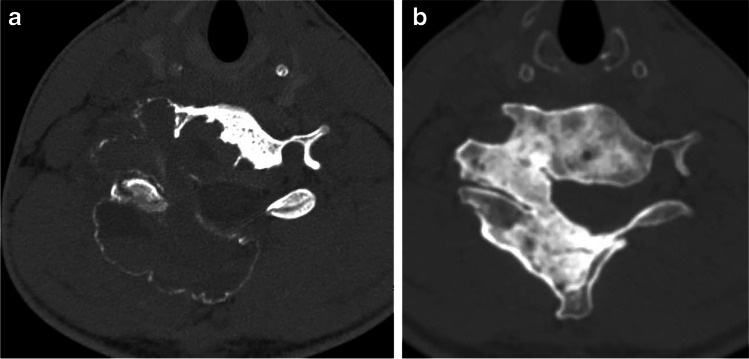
Computed tomography images in a 16-year-old boy (patient 5) with an aneurysmal bone cyst (ABC) involving C5, C6 and C7. a Diagnostic axial CT at C6 level before first treatment shows ABC expanding and replacing the entire right half of the vertebral ring at that level. b Diagnostic axial CT at same level 5 years after last treatment shows sclerotic bone has replaced the entirety of the ABC. This appearance was similar at all the other levels
